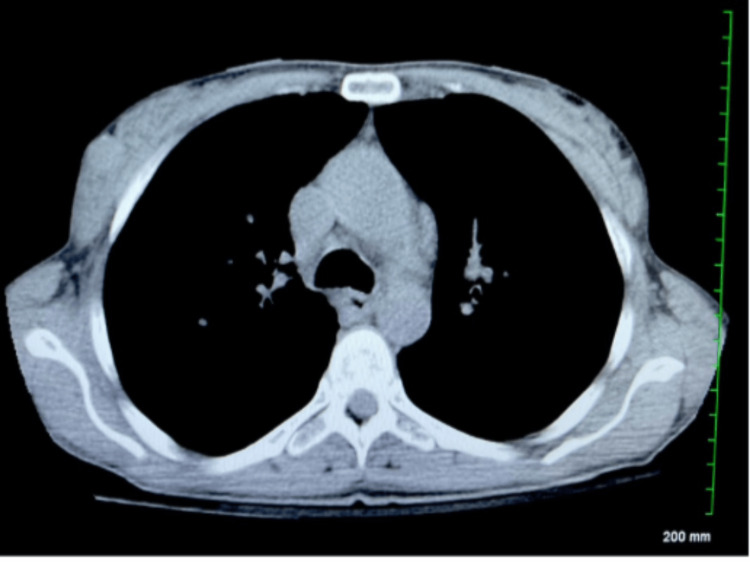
Initial chest CT angiogram without contrast CT: computed tomography
